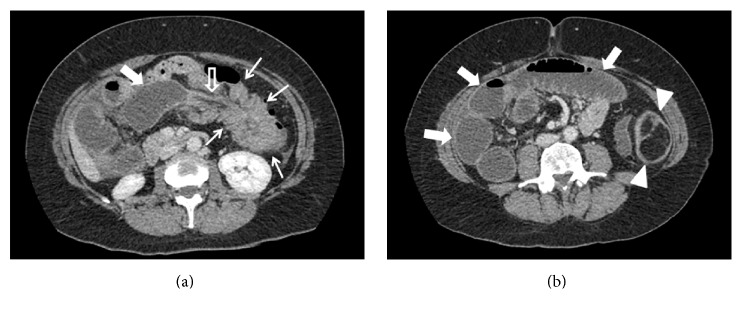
Contrast-enhanced CT, portal venous phase, axial images. In (a) the last ileal loop is dilated (full arrow); a part of the right colon and its mesentery (empty arrow) are recognizable inside the left colon (thin arrows). In (b) the section is at a lower level; on the left side, the colonic lipoma (arrowheads) is recognizable as a lobulated lesion with fat-equivalent density and with inner septa. On the right side notice dilated small bowel loops (full arrows) and absence of the right colon.
